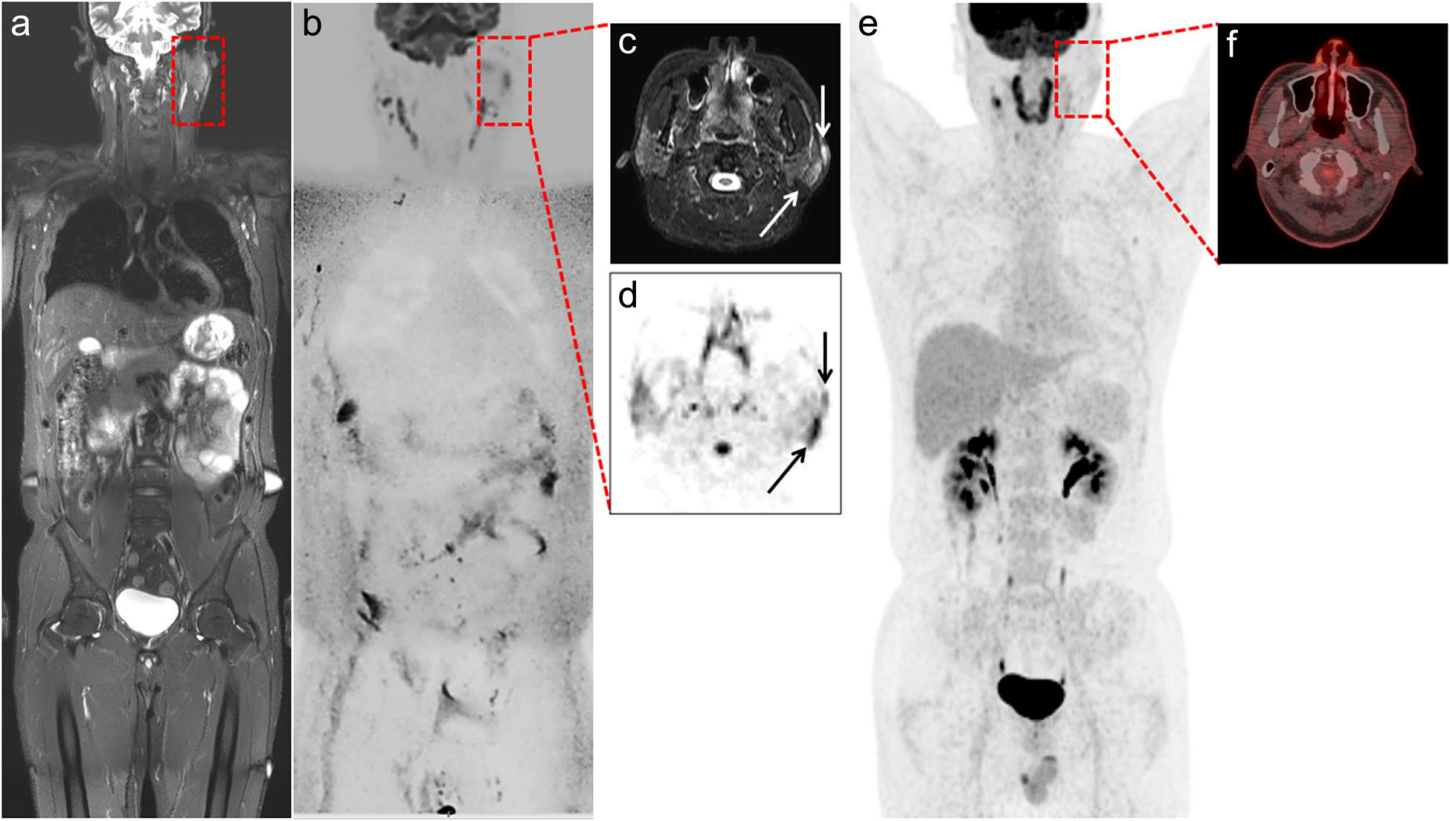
A 43-year-old man with MALToma of pretherapeutic stage IV. a Coronal whole-body T2-STIR, b DWIBS, c axial T2-STIR, d and axial DWIBS show lymphoma involvement in the left parotid gland and overlying skin (dashed square and arrows). e Coronal whole-body ^18^F-FDG-PET/CT MIP and (f) ^18^F-FDG-PET/CT axial fusion images do not show 18F-FDG uptake in the corresponding area. DWIBS = diffusion-weighted imaging with background body signal suppression, T2-STIR = T2-weighted short-tau inversion recovery, ^18^F-FDG-PET/CT = 18F-fluorodeoxyglucose-positron emission tomography-computed tomography, MIP = maximum intensity projection
